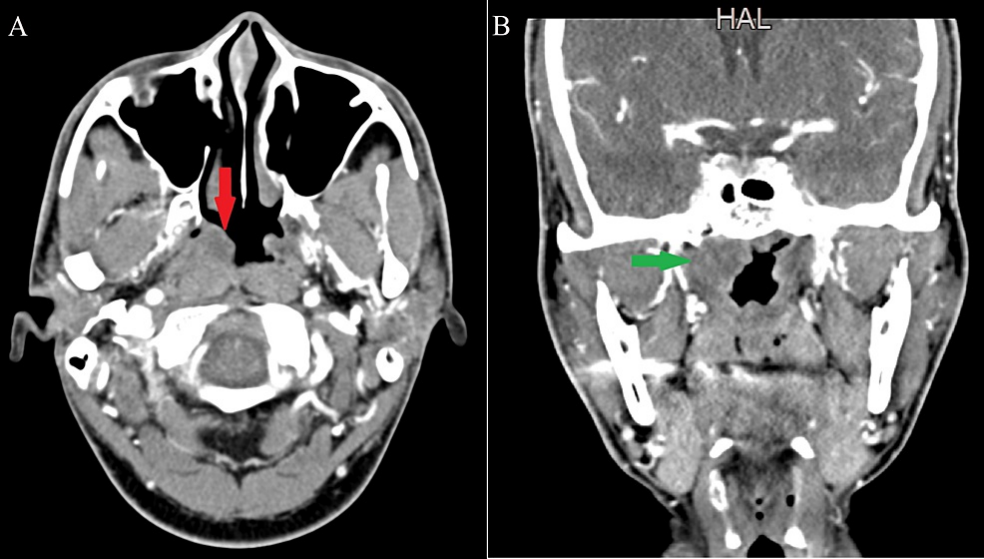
Computed tomography scan with contrast, A) axial cut showing right nasopharyngeal mass obliterating fossa of Rosenmuller (Red Arrow). B) coronal cut showing right nasopharyngeal mass obliterating fossa of Rosenmuller (Green Arrow)
